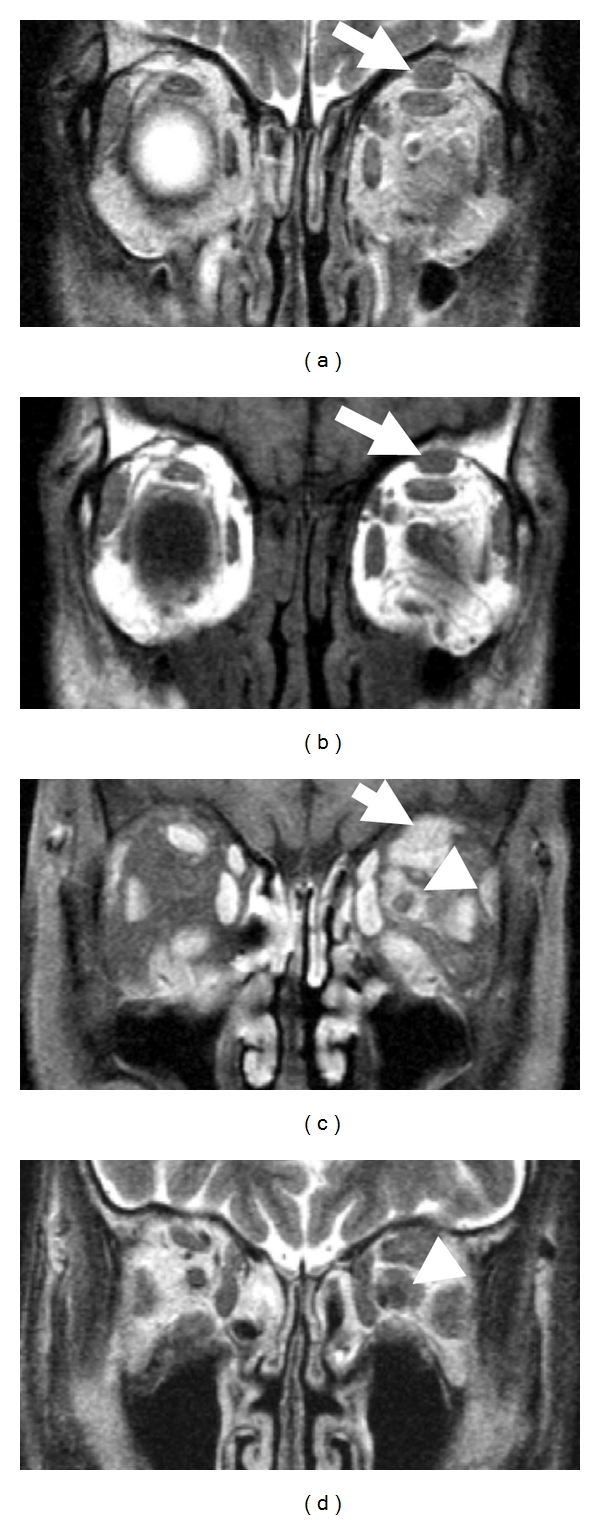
MRI ((a and d): T2-weighted images; b: T1-weighted image; c: contrast-enhanced T1-weighted image) of orbital space in 61-year-old man (case 3). MRI shows the soft tissue around the left supraorbital nerve (a–c; arrows) and optic nerve (c and d; arrowheads). The left optic nerve can be detected in the lesion.
